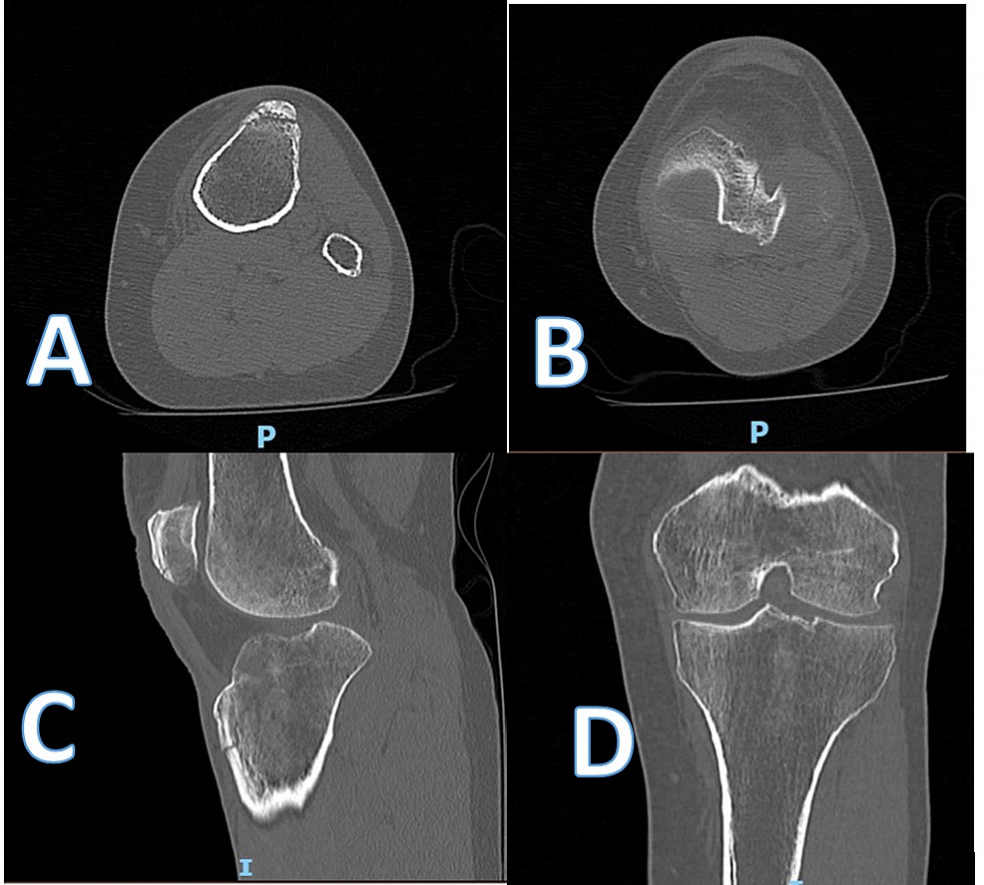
Axial (A,B), sagittal (C) and coronal (D) images of pre-operative CT scan of the left knee.
